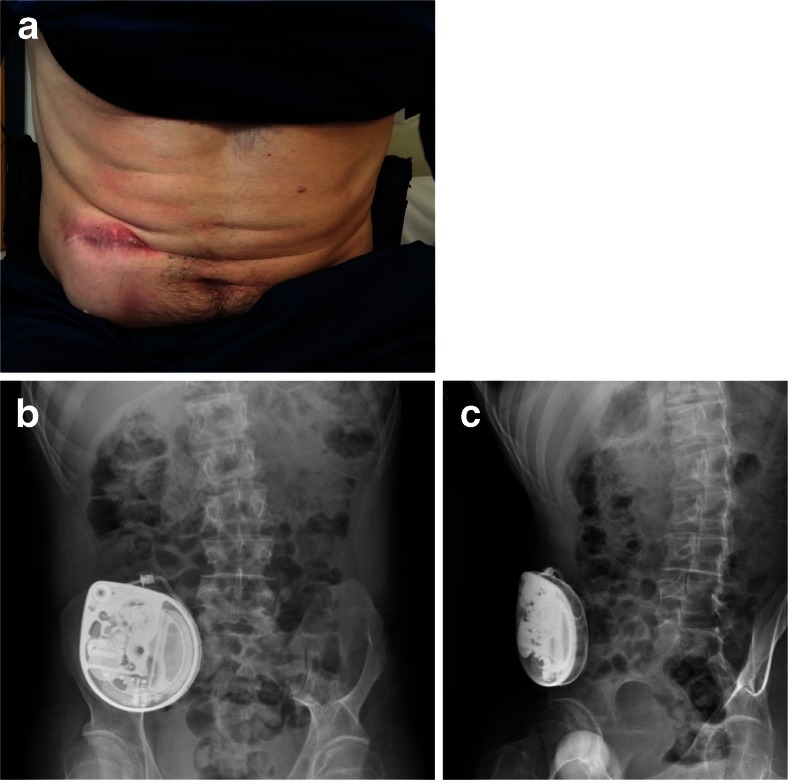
a Dehiscence of scar overlying the original site of intrathecal baclofen pump placement, 4 years after initial surgery. b Erect abdominal radiograph showing the original intrathecal baclofen pump device can be seen in the right lower quadrant of the abdomen with its catheter tracking laterally and posteriorly towards the right flank and onwards to the spinal column. c Lateral view abdominal radiograph depicting the paucity of subcutaneous tissue overlying the original pump site in the right lower quadrant of the abdomen
